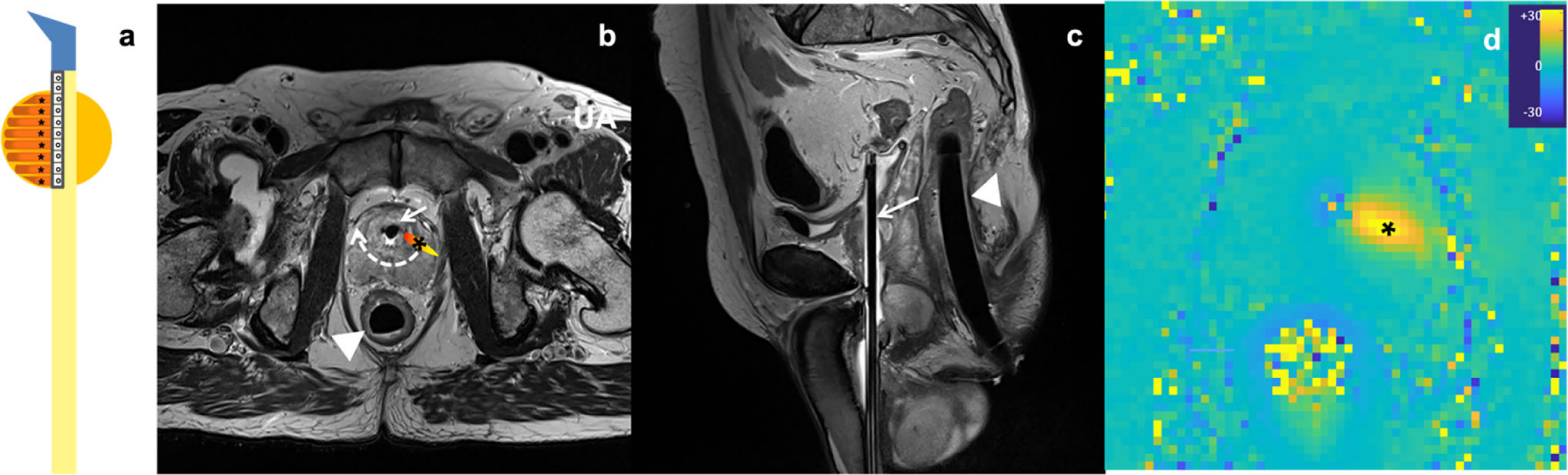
MRI-guided TULSA is a minimally invasive ablation technique providing thermal-mediated destruction of the prostate. a The rigid rotational transurethral ultrasound applicator delivers a directed, non-focused ultrasound energy (*) to the prostate (yellow circle) via ten independent ultrasound transducers (°). b, c Axial and sagittal T2W images show in situ position of transurethral ultrasound applicator (white arrow) and endorectal cooling device (white arrowhead). Dashed white arrow indicates the rotational sweep of directional ultrasound energy (*). d Real-time MR thermometry is continuously acquired to provide a prostatic temperature map, which is fed back to a dedicated algorithm to automatically adjusts the frequency and power of each UA transducer
